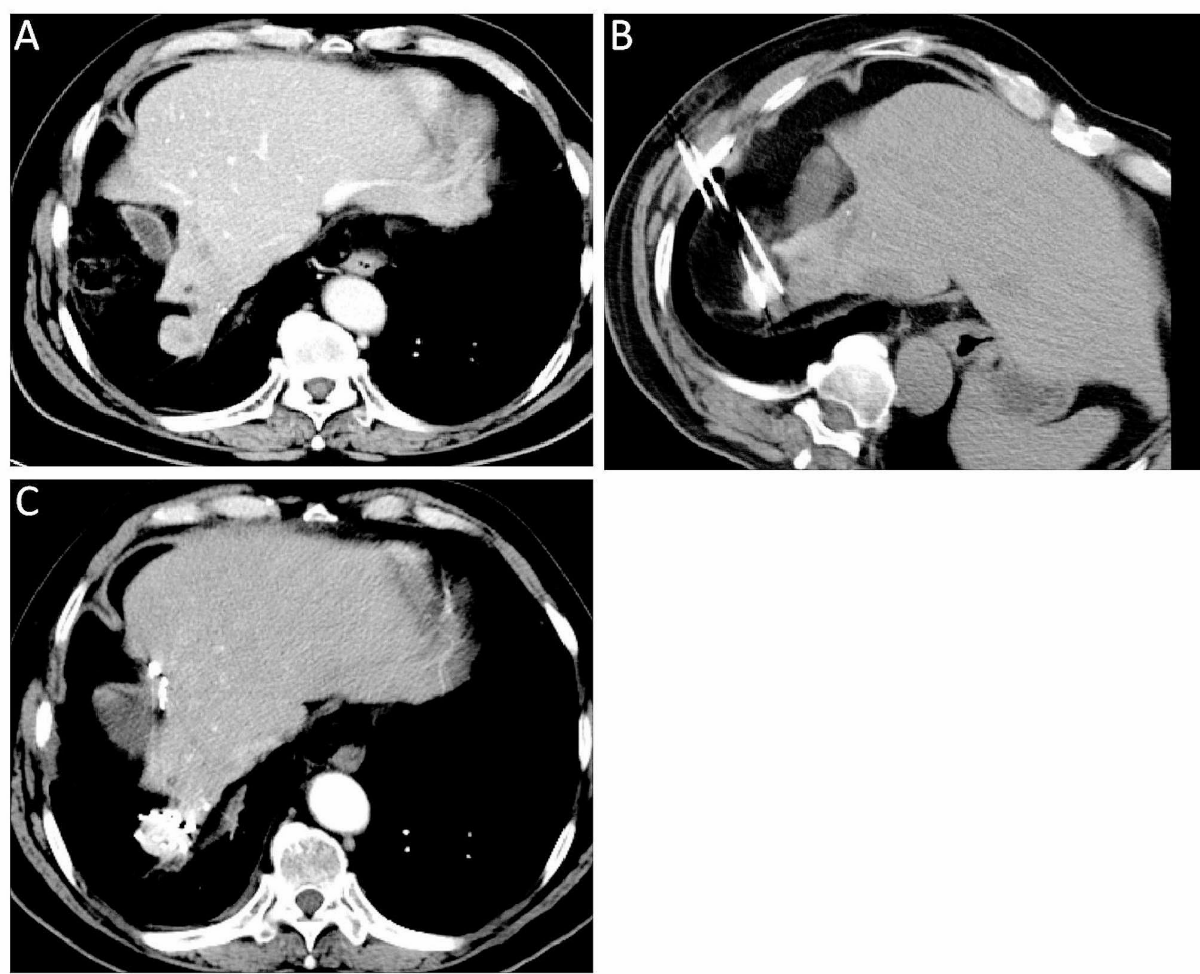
A 58-year-old male patient with HCC was diagnosed with recurrence 3 months after surgical resection. (A) Enhanced CT showed a 1.5 × 1.3 cm tumor in the right lobe of liver. (B) Immediately after RFA, the patient received I^125^ seeds implantation therapy; (C) CT reexamination 1 month later showed complete response according to the modified Response Evaluation Criteria in Solid Tumors
